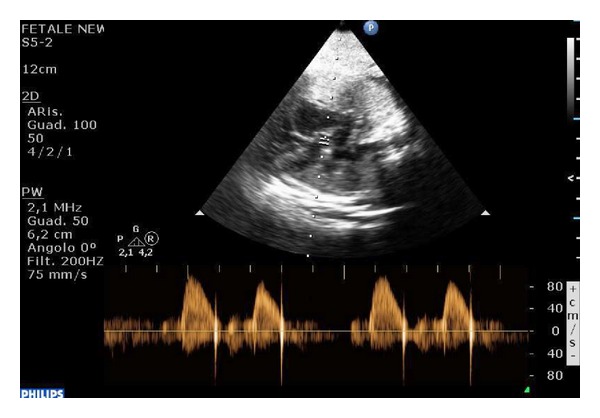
Pulsed Doppler wave of left ventricular outflow tract. There is a pause after 2 regular beats.
